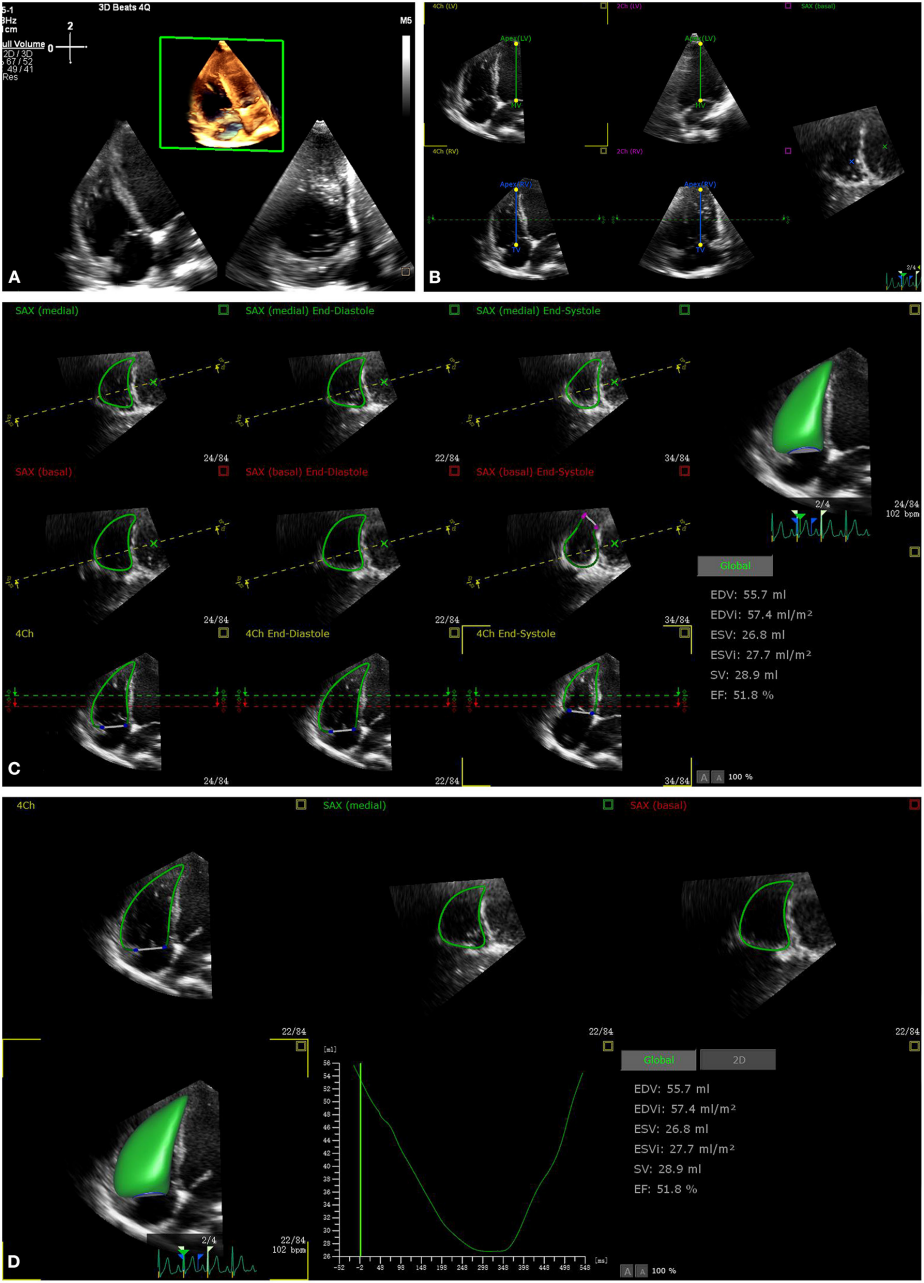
The process of fully automated right ventricular (RV) analysis. After retrieving the RV focus three-dimensional echocardiography (3DE) data aiming for RV analysis (A), start the software, automatically adjust the five landmarks (B), automatically determine the RV border at end diastole and end systole (C), and provide the results within 15 s (D).
